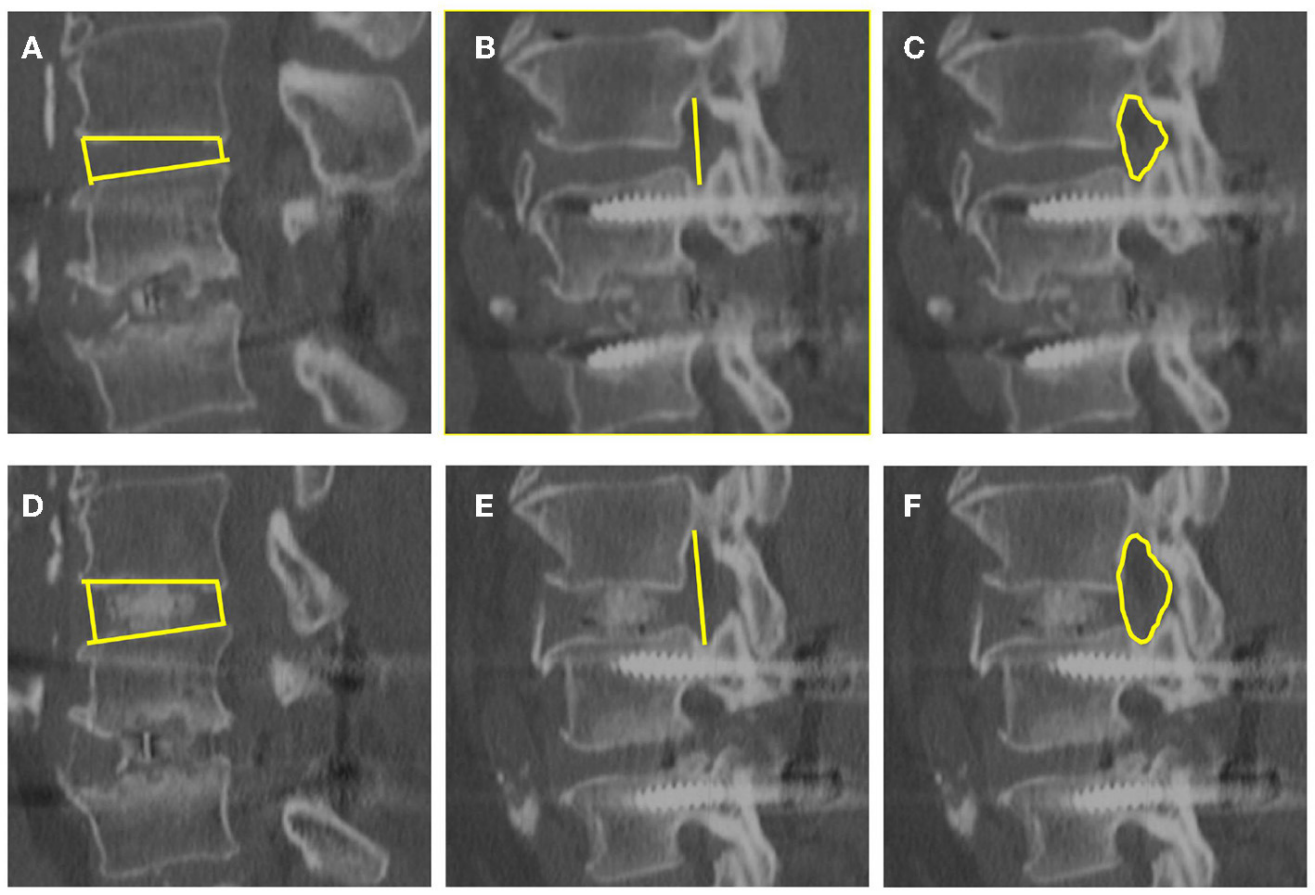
Images obtained in the same patient. Disk height (A,D), foraminal height (B,E), and foraminal CSA (C,F) were evaluated with CT before and at last follow-up after surgery. (A–C) are before surgery, and (D–F) are at last follow-up after surgery.
